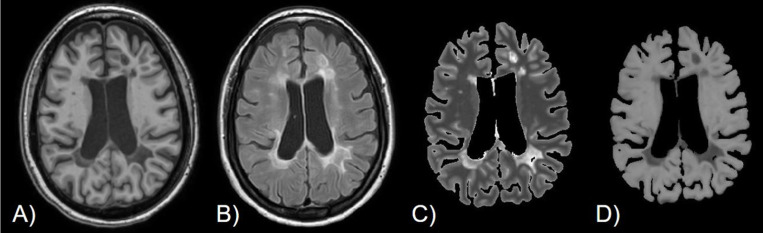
Representative depiction of: A) T1-weighted B) T2 FLAIR, C) retroactive T1 map, and D) MTR map.
